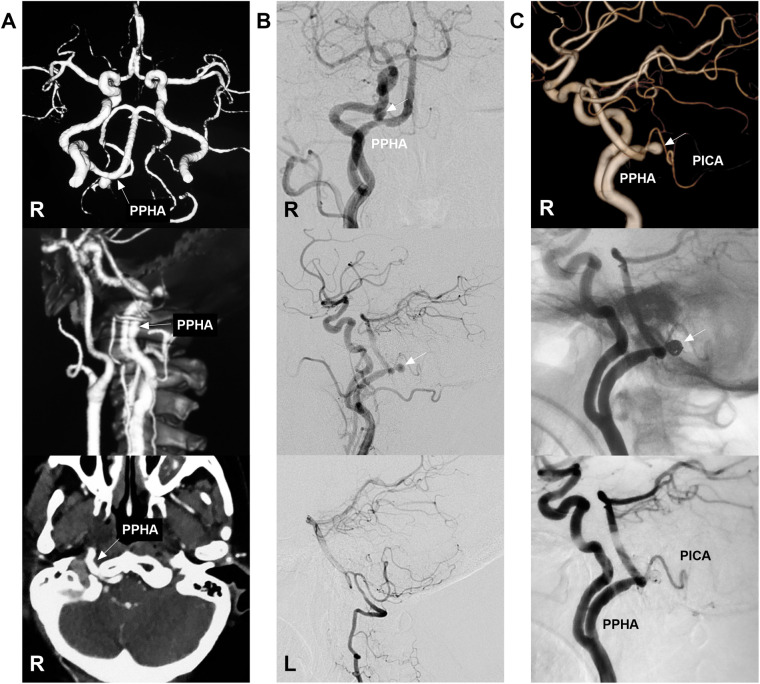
Coiling an aneurysm at the junction of PPHA and PICA. (A) Upper and middle panels: CTA (upper) and CTA with semi-transparent bone reconstruction (middle) demonstrate a right PPHA (arrows) extending into the posterior cranial fossa. Lower panel: Maximum intensity projection of CTA reveals the PPHA (arrow) coursing through the hypoglossal canal. (B) Upper and middle panels: DSA images of the right carotid artery in anteroposterior (upper) and lateral (middle) views show an aneurysm at the junction of the PPHA and PICA (arrows). Lower panel: DSA of the left VA demonstrates a hypoplastic VA. (C) Upper panel: Three-dimensional DSA displays the saccular aneurysm (arrow). Middle panel: Unsubtracted DSA confirms the aneurysm (arrow) was coiled via the PPHA. Lower panel: Post-procedural DSA confirms patency of the PPHA and PICA. CTA, computed tomography angiography; DSA, digital subtraction angiography; L, left; PICA, posterior inferior cerebellar artery; PPHA, persistent primitive hypoglossal artery; R, right; VA, vertebral artery.
